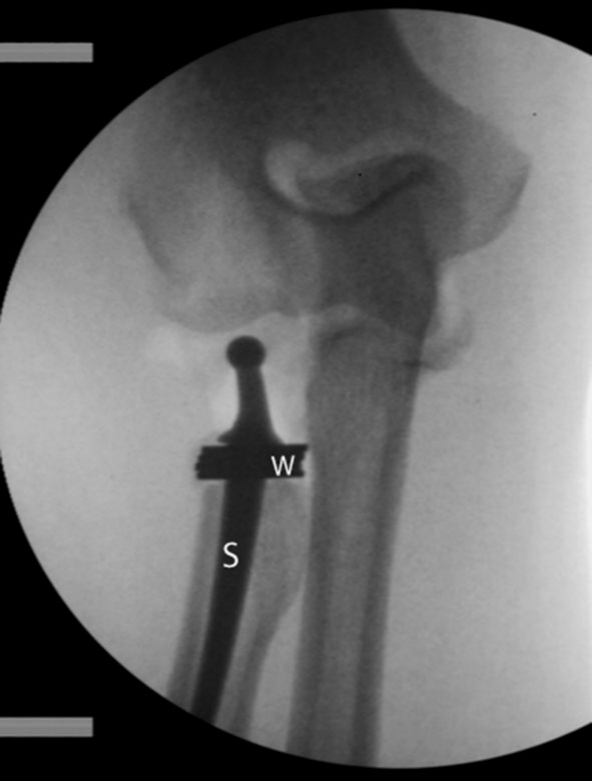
X-ray image, showing a multipolar prosthesis with an artificial overlengthening of +6 mm. The head of the prosthesis is radiolucent, as a trial head component was used. *S* radial shaft; *W* metal washers
